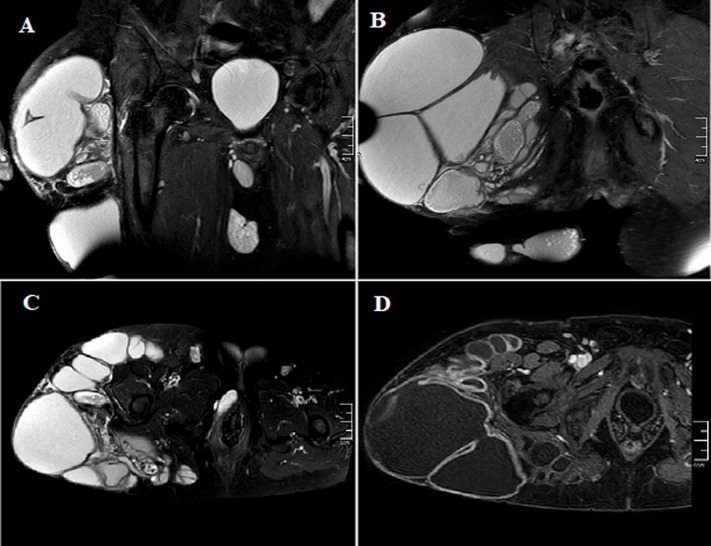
A) coronal T2-weighted image; B,C) axial T2-weighted images, coronal T1-weighted image with gadolinium injection; D) multiple cystic formations on the posterolateral aspect of the right thigh root and gluteal region, occupying mainly subcutaneous fat, gluteus maximus muscle and subaponeurotic fat of the hamstring compartment; they have a thin wall in T2 hyposignal enhanced after injection of gadolinium and fluid content of a variable signal, some of which are multiloculated and septated
